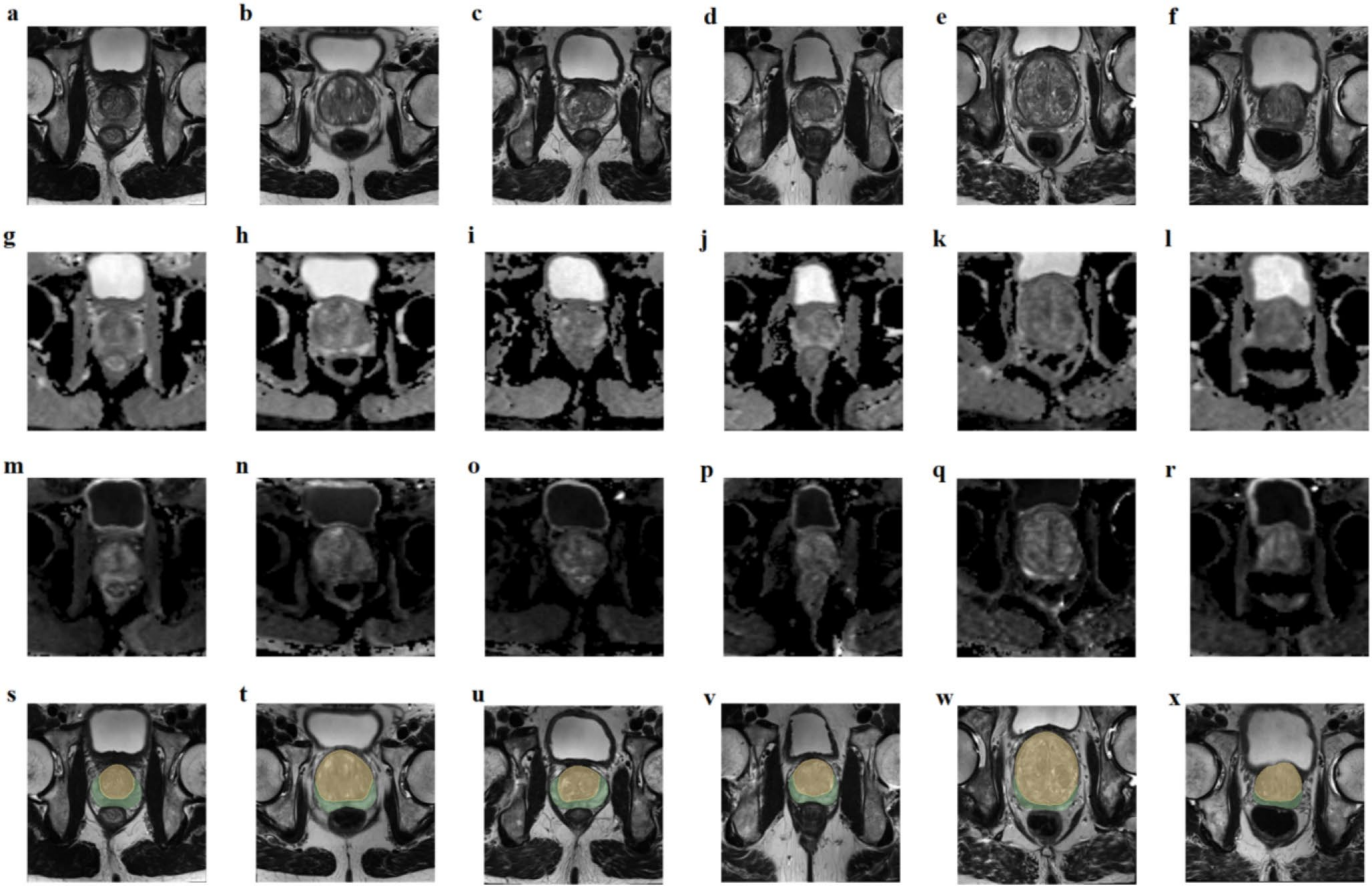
Examples T2WI, ADC and DWI images and masks on T2WI in PZ/CG: ISUP 0 of T2WI (a), ISUP 1 of T2WI (b), ISUP 2 of T2WI (c), ISUP 3 of T2WI (d), ISUP 4 of T2WI (e), ISUP 5 of T2WI (f), ISUP 0 of ADC (g), ISUP 1 of ADC (h), ISUP 2 of ADC (i), ISUP 3 of ADC (j), ISUP 4 of ADC (k), ISUP 5 of ADC (l), ISUP 0 of DWI (m), ISUP 1 of DWI (n), ISUP 2 of DWI (o), ISUP 3 of DWI (p), ISUP 4 of DWI (q), ISUP 5 of DWI (r), segmentation on T2WI of ISUP 0 (s), ISUP 1 (t), ISUP 2 (u), ISUP 3 (v), ISUP 4 (w), and ISUP 5 (x)
